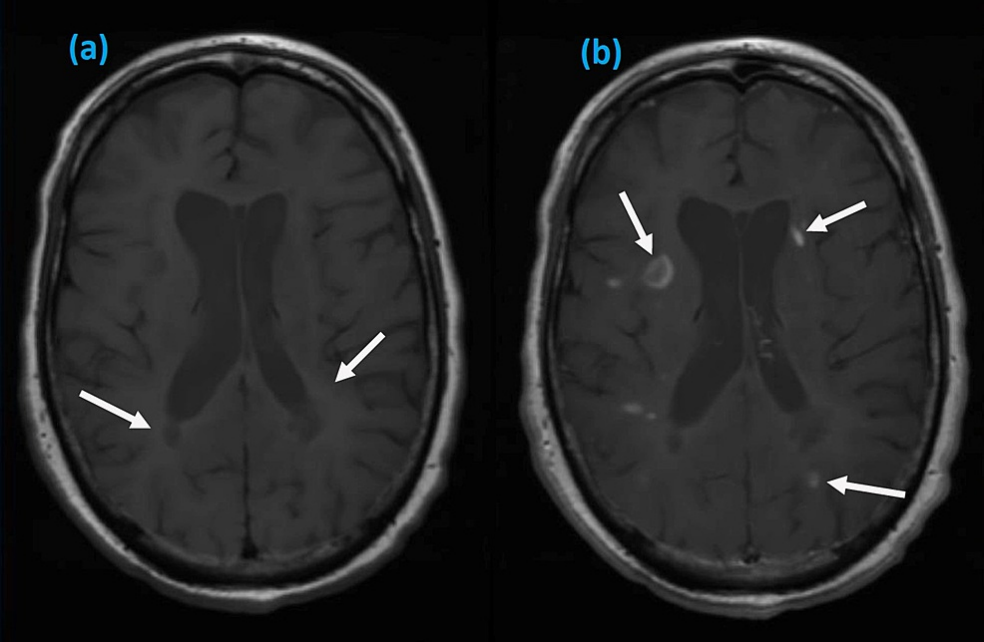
Axial T1 MRI images showing periventricular lesions of various intensities in posterior (a, b) and parietal lobe (b) MRI: magnetic resonance imaging
